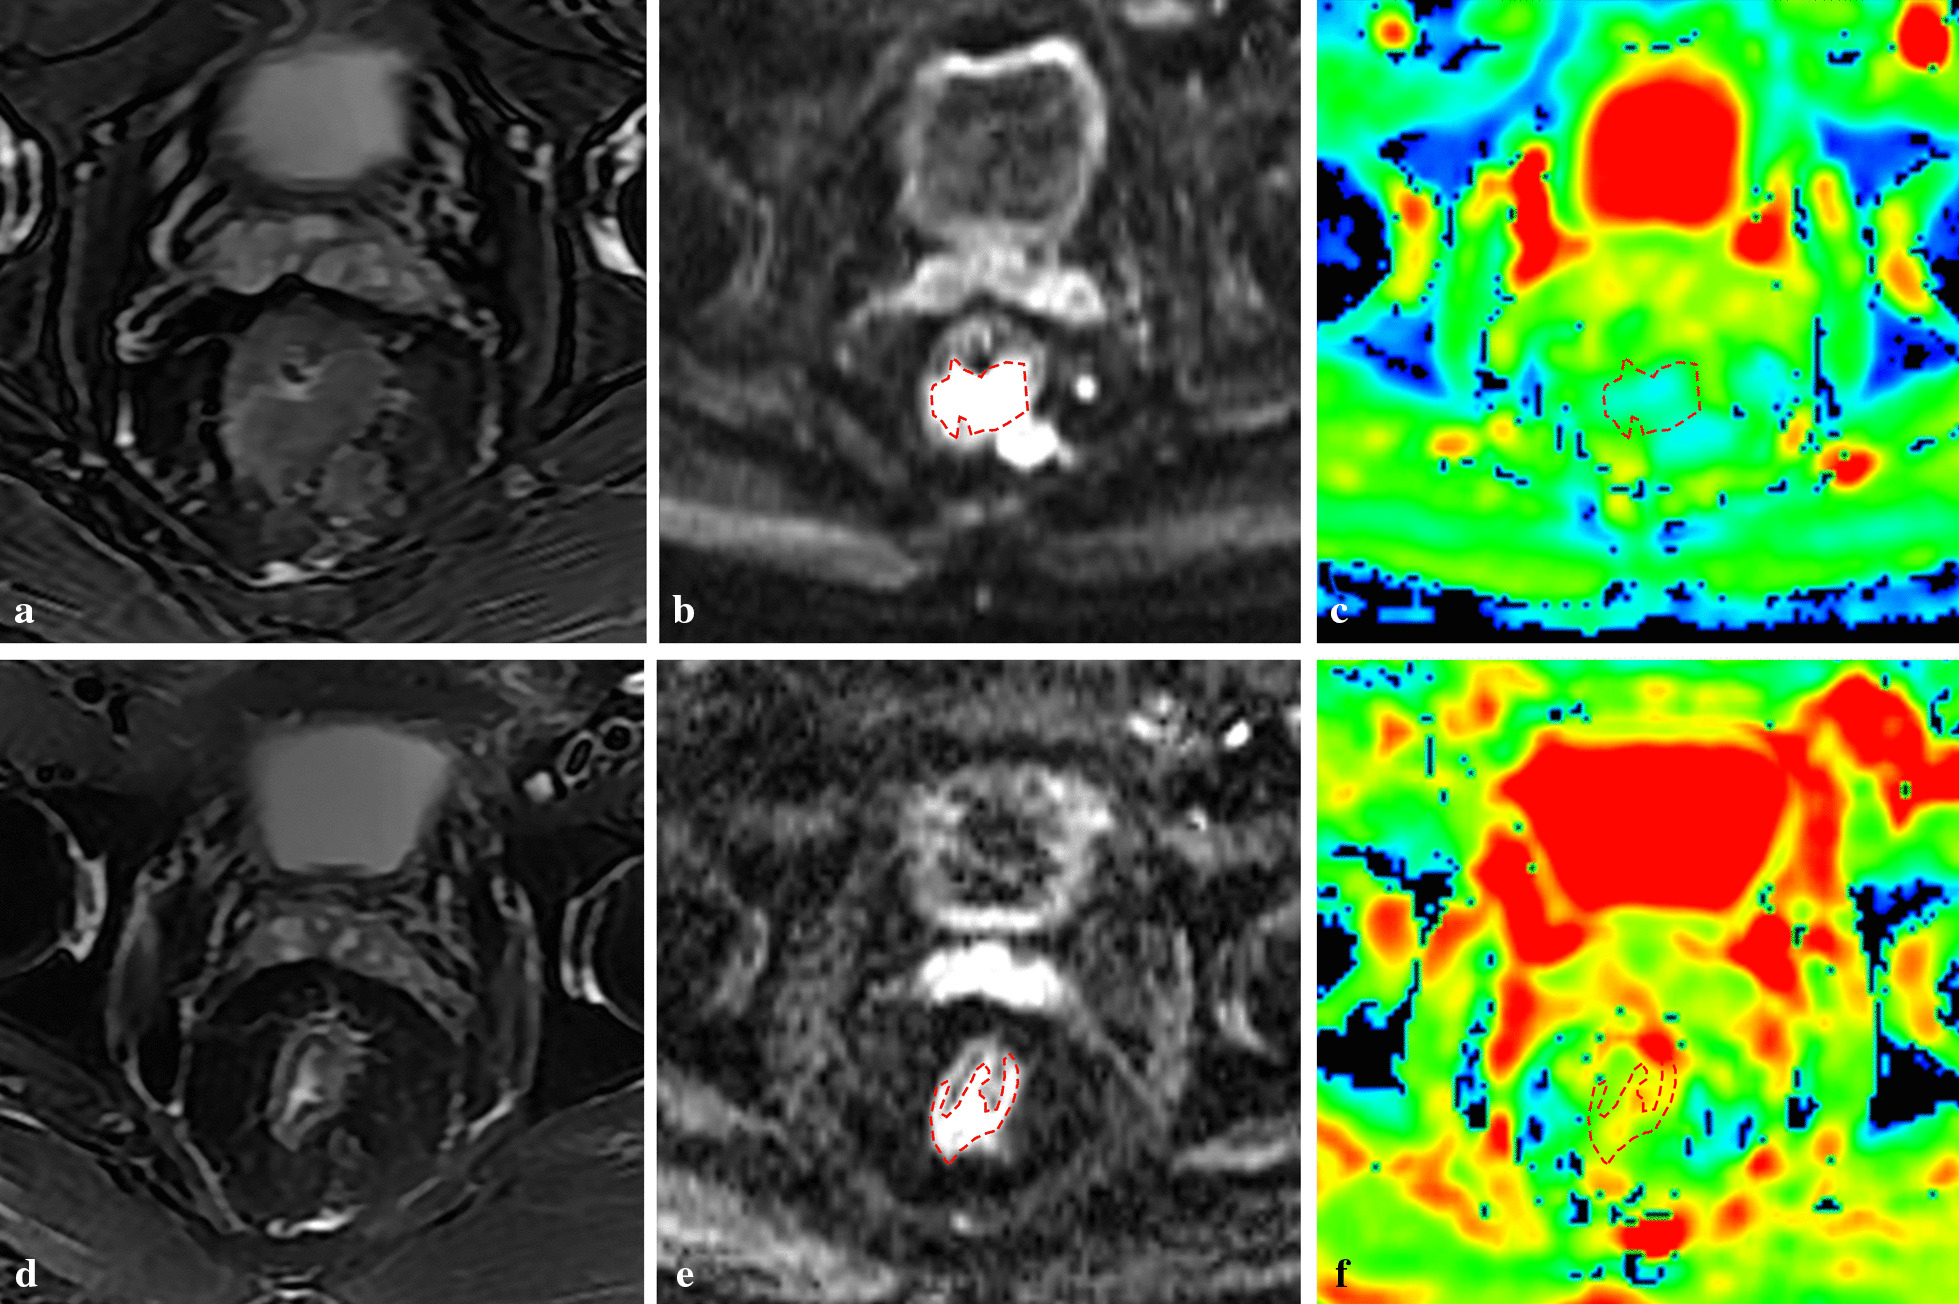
MRI change of rectal cancer before and after treatment of neoadjuvant chemo-radiotherapy. a T2WI STIR before treatment of neoadjuvant chemoradiotherapy; b DWI before treatment of neoadjuvant chemoradiotherapy; c ADC map before treatment of neoadjuvant chemoradiotherapy; d T2WI STIR after treatment of neoadjuvant chemoradiotherapy; e DWI after treatment of neoadjuvant chemoradiotherapy; f ADC map after treatment of neoadjuvant chemoradiotherapy;
